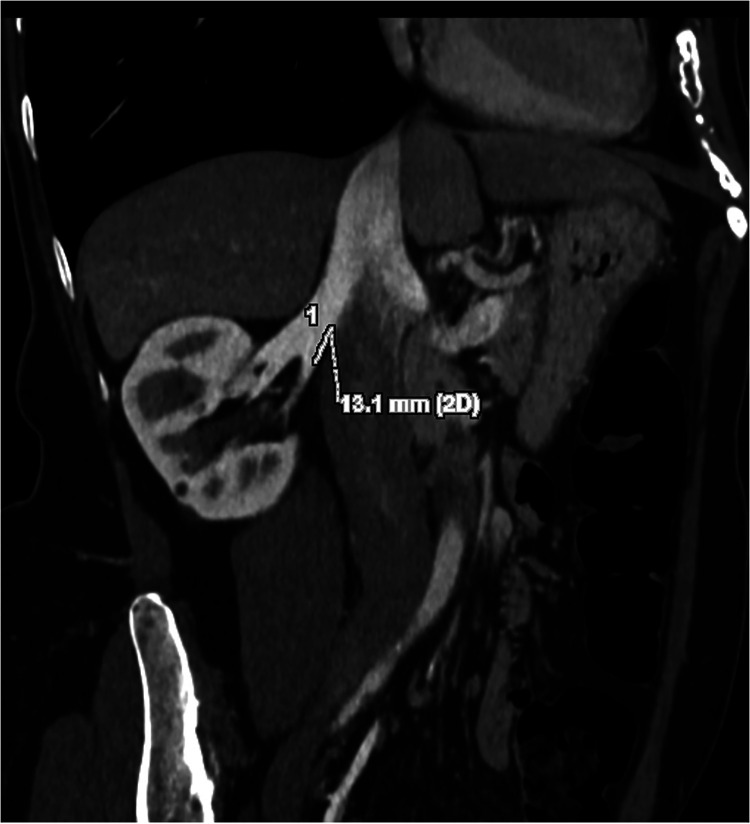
Preoperative donor CT scan (multiplanar coronal-oblique reconstruction related to case #7 in Table 1). The right renal vein measures 13 mm from the caval ostium to the hilar division.
